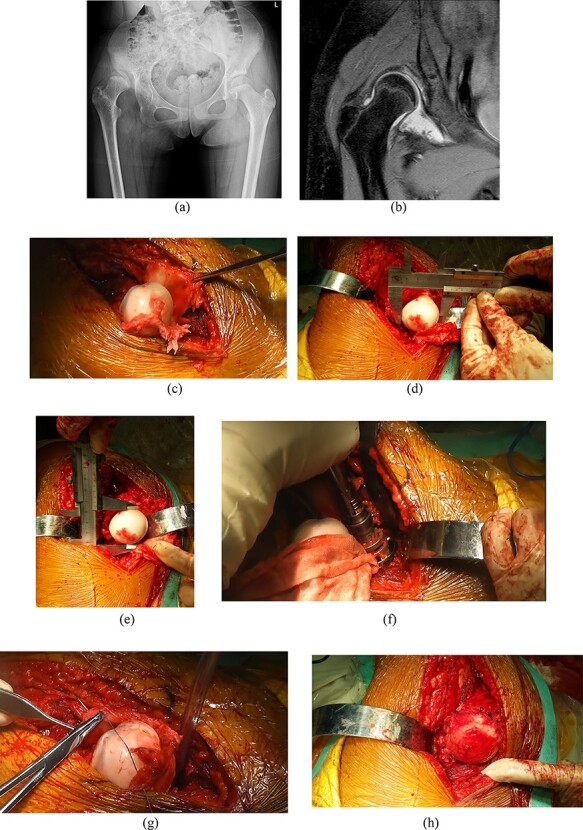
The modified capsular arthroplasty in a 13-year-old female patient with DDLH (iHot 62.3, WOMAC 9 and mHHS 74). (A) Pre-operative anterior-posterior radiograph shows the Hartofilakidis Type III dislocation of the hip. (B) Pre-operative MRI shows the good quality of capsular. The outermost superior–lateral capsular thickness is 5.3 mm. (C–H) Intraoperative pictures show the brief steps of the surgery. (C) The femoral head is dislocated and all capsular connections with the acetabular rim are sectioned. (D) and (E) show the measurements of anteroposterior and medial–lateral femoral head diameters. (F) shows the reaming of the acetabular socket. (G) and (H) show the suture of the capsule over the head. X-ray immediately after surgery (I), 2 years post-operatively (J) and 6 years post-operatively (K) (iHot 91.1, WOMAC 5, mHHS 94).
